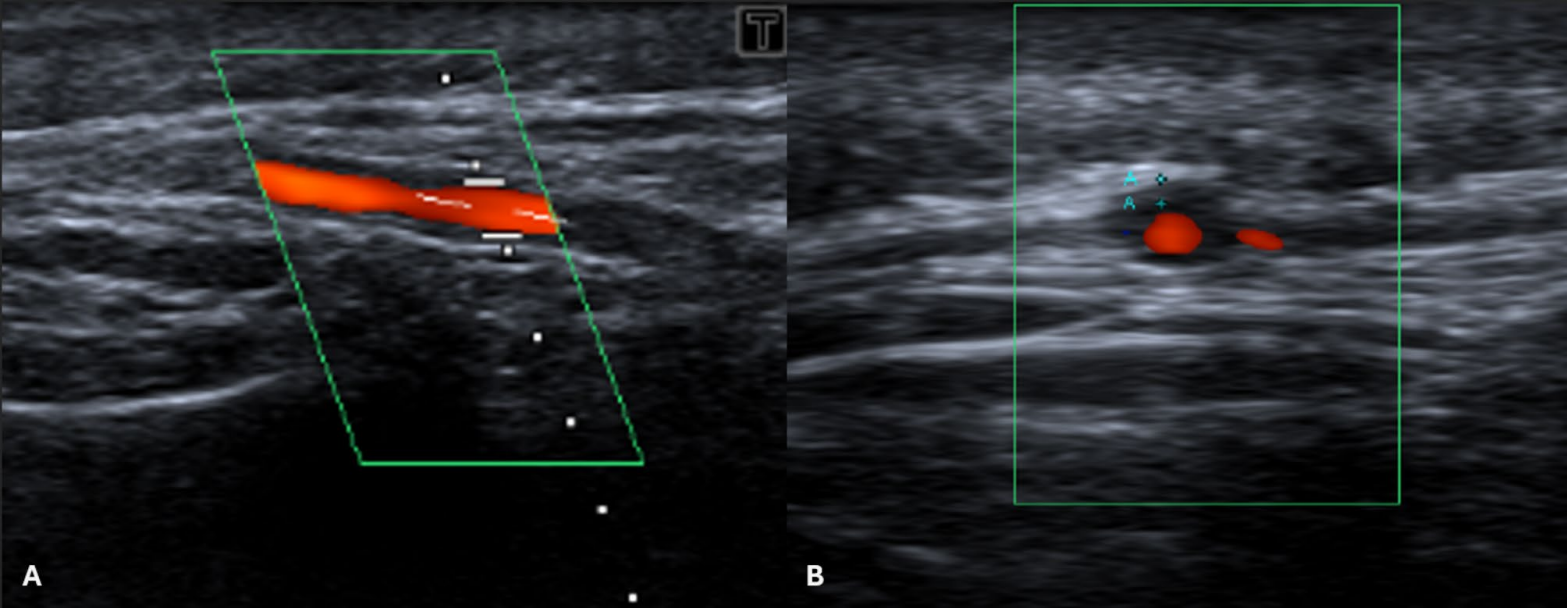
Duplex ultrasound of the right temporal artery. (A) Longitudinal view with visible thickening of the vessel wall. (B) Transverse view with halo sign indicative of GCA
